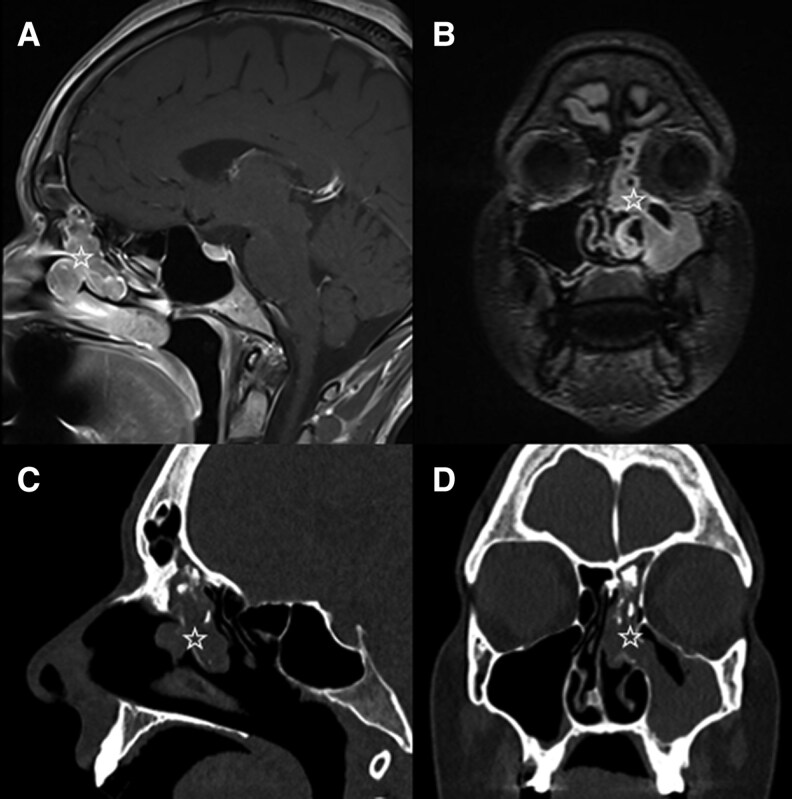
The T2-weighted sequence of a sagittal (A) and coronar (B) post-contrast MRI demonstrates a contrast-enhancing mass in the left maxillary sinus, extending into the sphenoid and frontal sinuses, with diffuse swelling of the middle and superior nasal conchae (star). A sagittal (C) and coronar (D) non-contrast CT scan shows intrasinusal and intraductal expansion (star) with destructive bone alterations.
